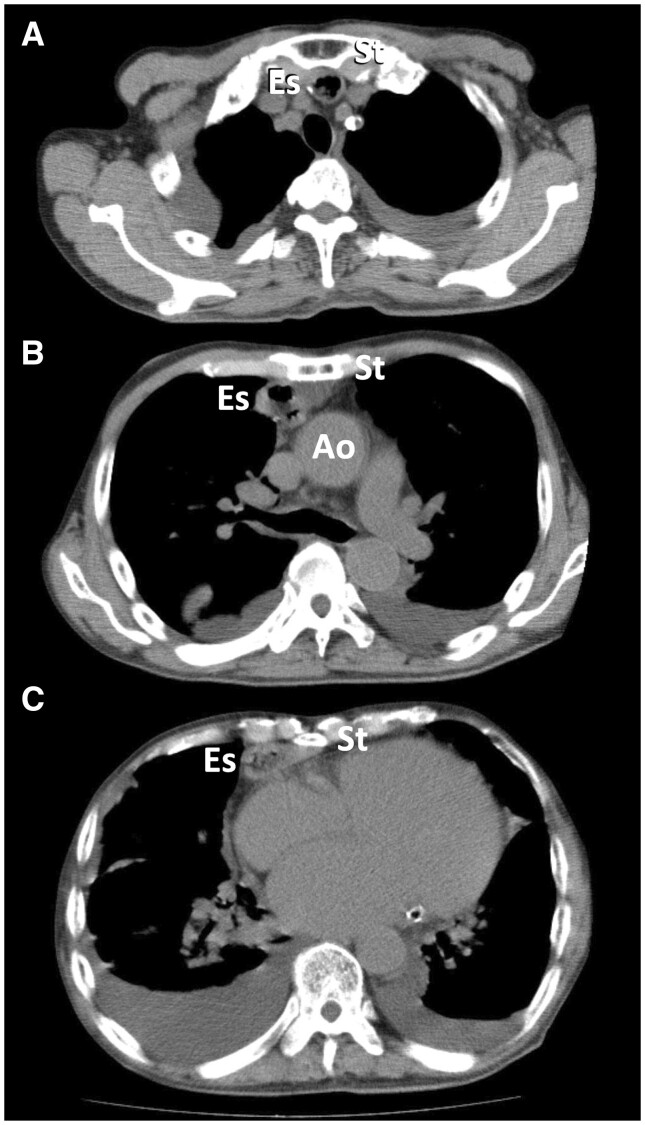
Computed tomography showing relationship among the reconstructed oesophageal conduit (Es), sternum (St) and ascending aorta (Ao) at the level of the (a) upper, (b) middle and (c) lower parts of the sternum.
